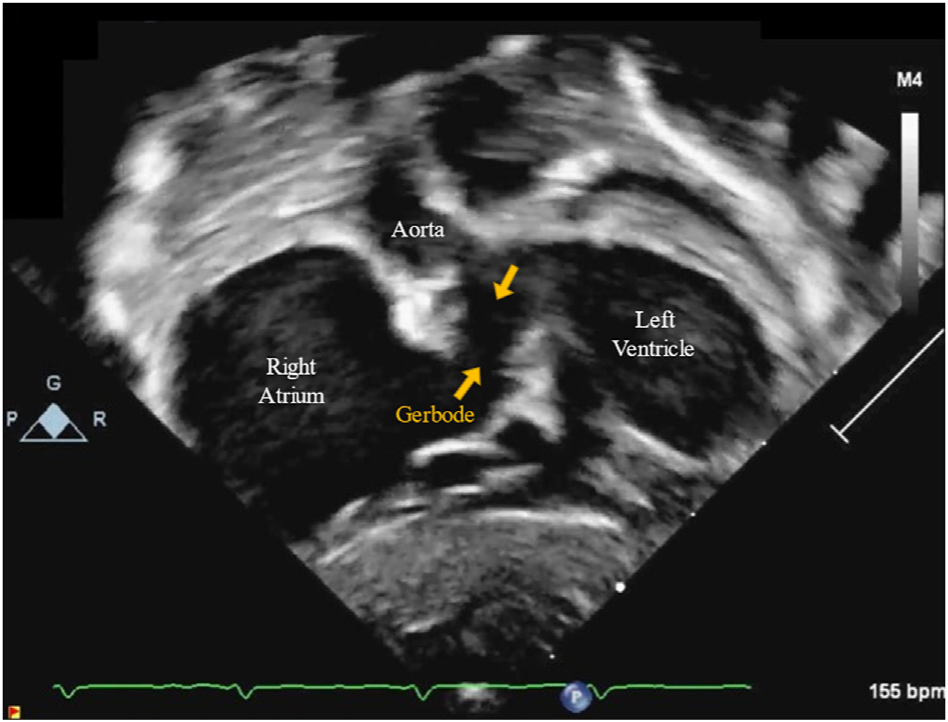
Transthoracic echocardiography demonstrating patient’s Gerbode defect (arrows).
